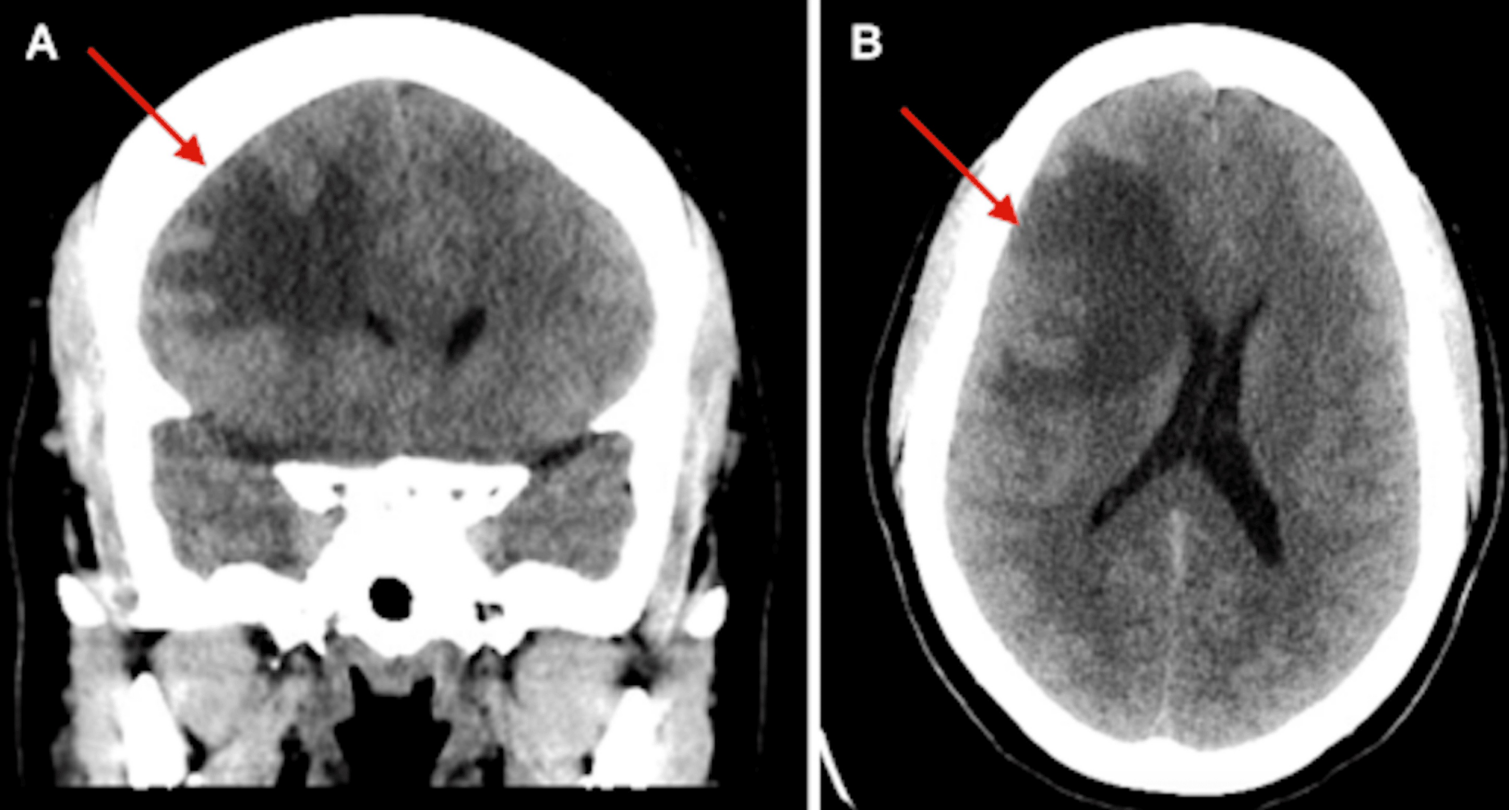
CT head showing a 3.6 cm mass (red arrows) within the right frontal lobe with associated vasogenic edema and mass effect on the right lateral ventricle. (A) Coronal view demonstrating mass effect on right lateral ventricle. (B) Axial view demonstrating 6 mm of leftward midline shift.
